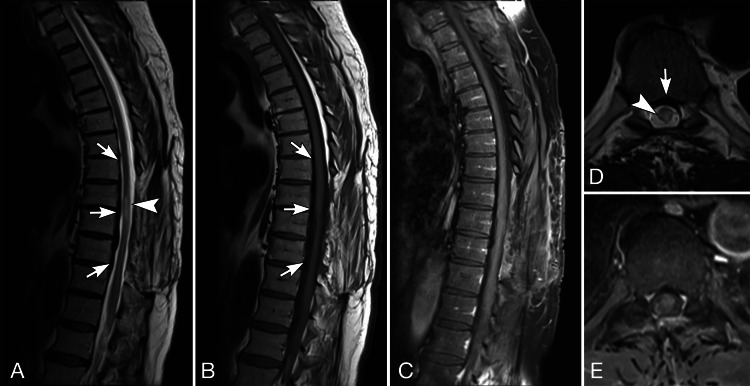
MRI of the thoracic spine 10 months after the prior thoracic MRI. Sagittal T2 (A) and T1- pre-contrast (B) and T1-weighted fat-suppressed post-contrast (C) MRI, and axial T2 (D) and fat-suppressed post-contrast T1-weighted (E) MRI. The axial images are at T8-T9. There has been further interval decrease in the T2 and T1-hypointense ventral dural lesion from T7-T11 (white arrows in A, B, D). The improvement includes resolution of mass effect on the spinal cord and resolution of enhancement. The spinal cord T2 hyperintensity has also improved in longitudinal extent, now spanning only slightly above and below the T8-T9 interspace (black arrows in A, D).
